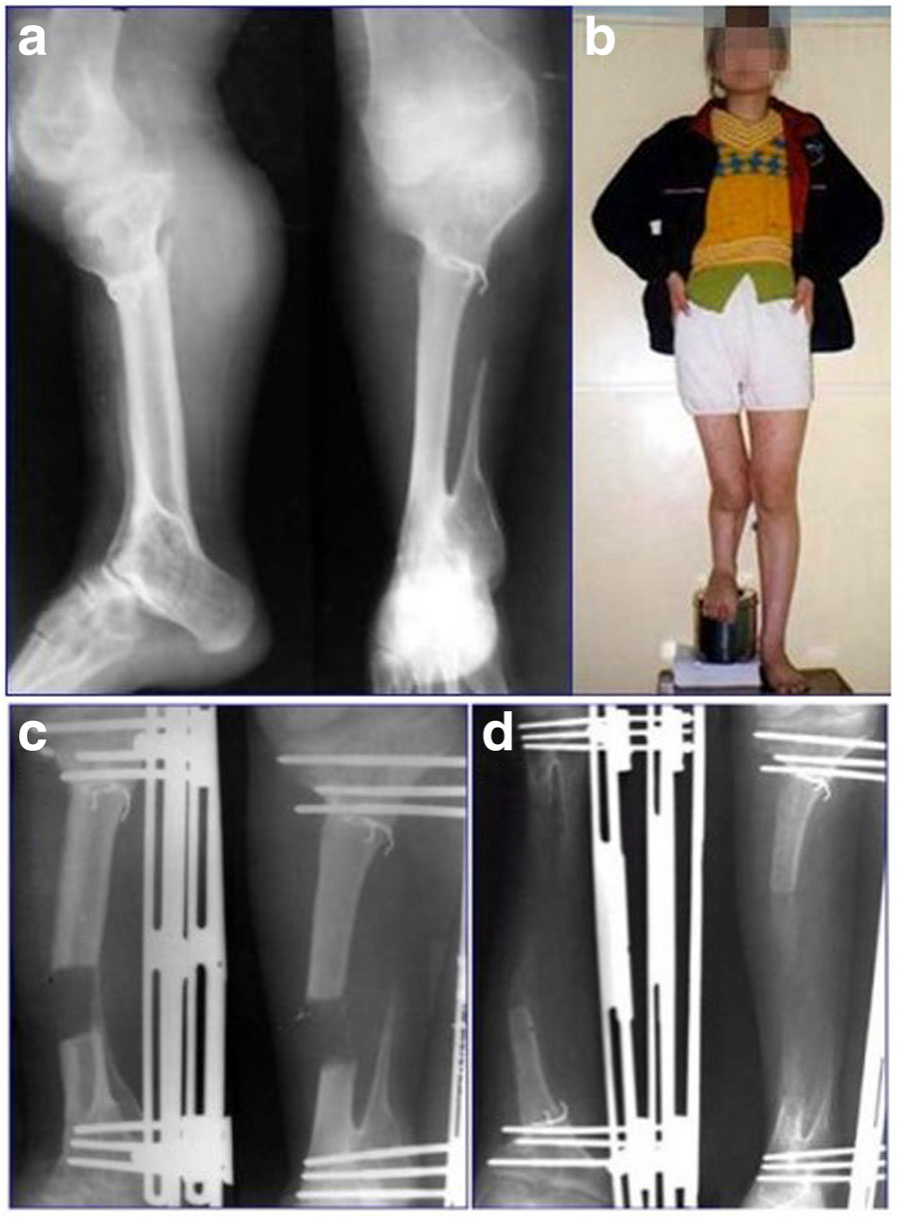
The X-ray film of case 1 reveals reconstruction of the tibia with a FVFG was done (a), she has 18 cm shortness at the right limb (b), the length correction program with unilateral external fixator was done and the callus regenerated well (c, d)
